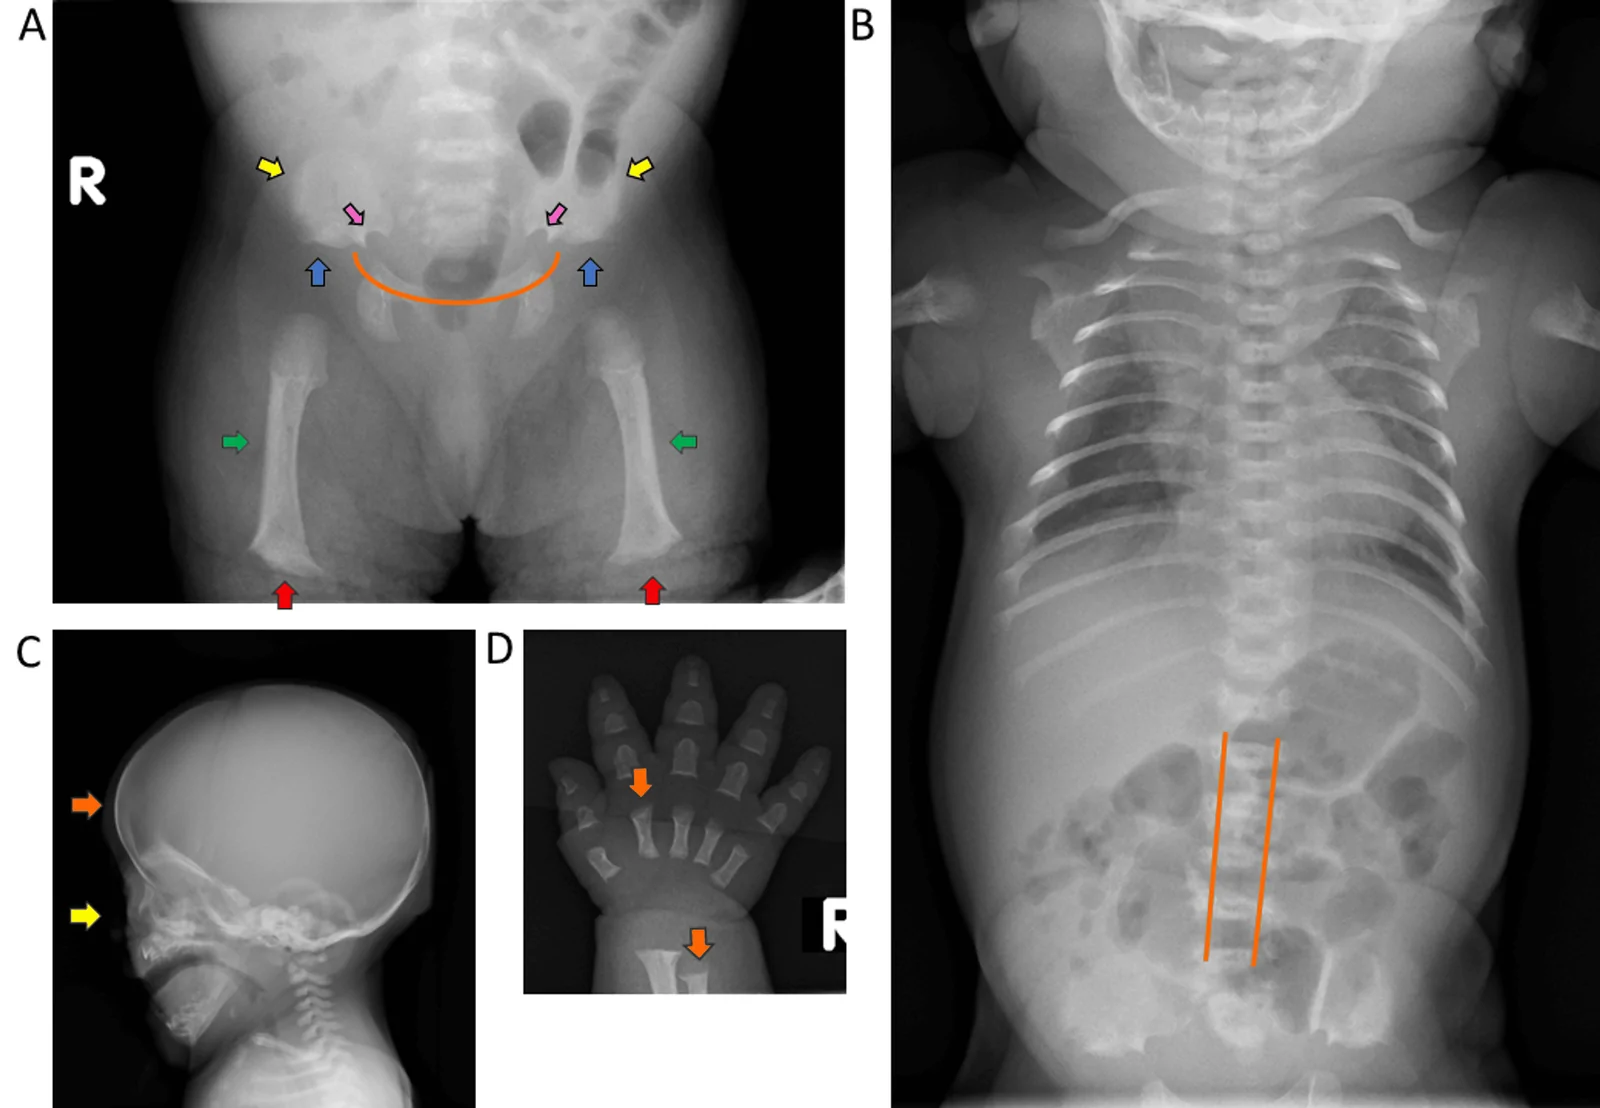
Radiographic findings in the neonatal period. (A) Pelvis and femurs: Anteroposterior view demonstrating a “champagne glass” pelvic inlet (orange line), squared iliac wings (yellow arrows), horizontal acetabular roofs (blue arrows), and narrow sacrosciatic notches (pink arrows). Pronounced rhizomelic shortening of the femurs (green arrows) is observed, accompanied by metaphyseal flaring and cupping (red arrows). (B) Spine: Anteroposterior view showing the interpedicular distance slightly narrowing from L1 to L5 (orange lines). (C) Skull: Findings include prominent frontal bossing (orange arrow) and midface hypoplasia (yellow arrow). (D) Right hand: Characteristic metaphyseal flaring and cupping of the tubular bones (orange arrows).
